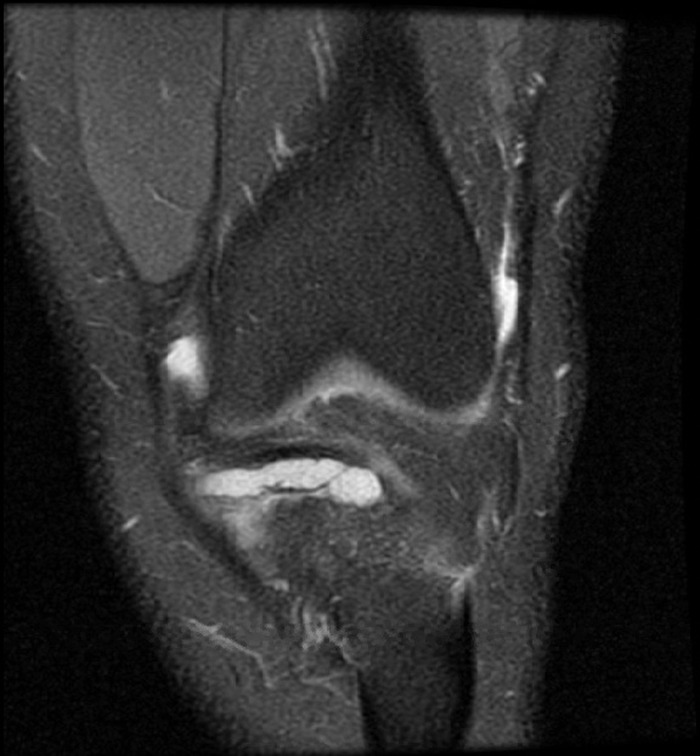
Coronal proton density fat-suppressed magnetic resonance image shows a right discoid medial meniscus measuring 3.58 × 0.74 cm.
